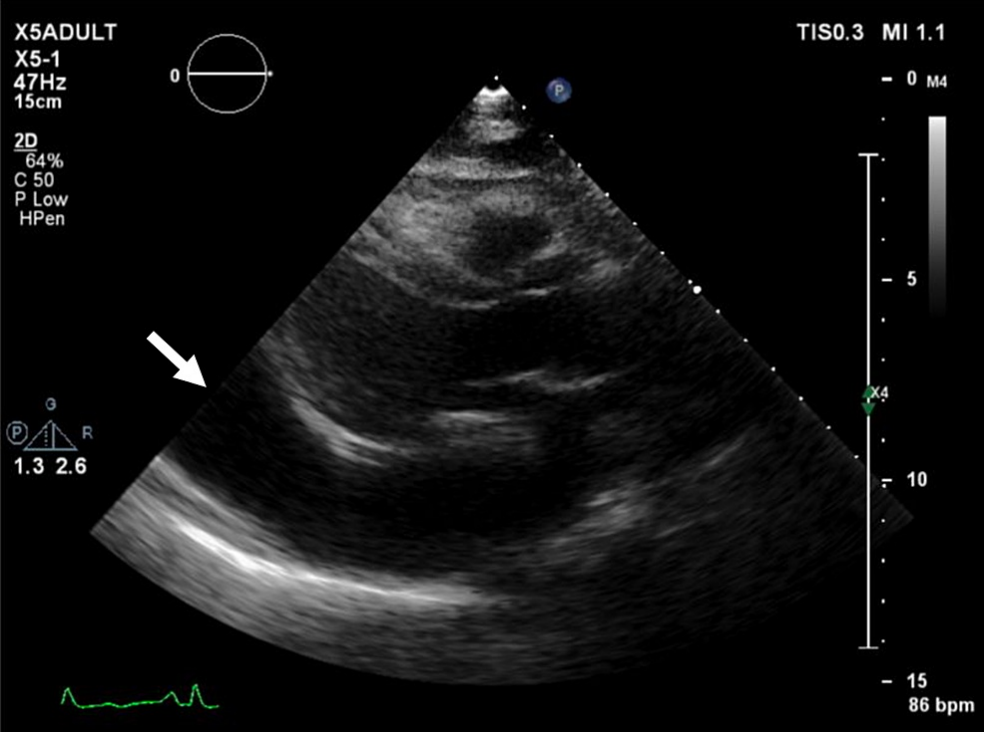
Two-dimensional echocardiography showed massive pericardial effusion (arrow) causing cardiac tamponade.
